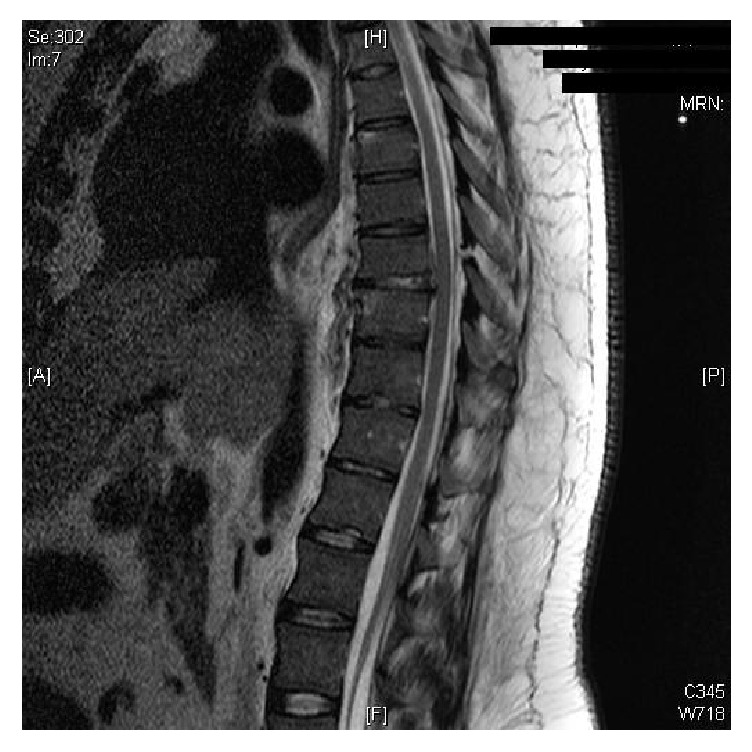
MRI of the thoracic spine on presentation to the ED, showing rupture of the ligamentum flavum at T7/8 and a hyperintense signal in the intervertebral disc at T8/9.
